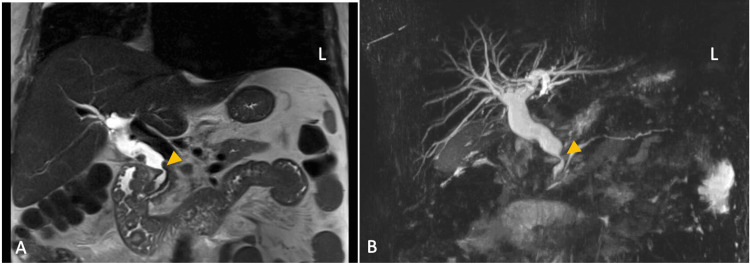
MRI abdomen (A) Coronal T2-weighted sequence showing a dilated CBD with abrupt cut-off (indicated by yellow arrowhead); (B) Coronal MIP demonstrating intrahepatic and extrahepatic biliary dilation with CBD stricture (indicated by yellow arrowhead). CBD, Common Bile Duct; MIP, Maximum Intensity Projection; MRI, Magnetic Resonance Imaging
